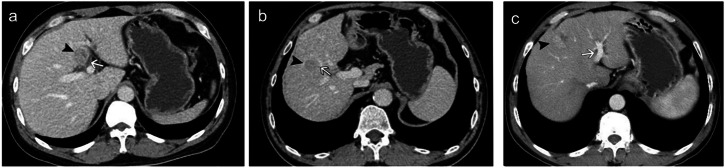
Images of peribiliary HCC (a, b) and non-peribiliary HCC (c). a Axial CT image of peribiliary HCC (black arrowhead) adjacent to the first-order branches of the intrahepatic bile duct (white arrow) with distance ≤ 5 mm. b Axial CT image of peribiliary HCC (black arrowhead) adjacent to the second-order branches of the intrahepatic bile duct (white arrow) with distance ≤ 5 mm. c Axial CT image of non-peribiliary HCC (black arrowhead) distal to the intrahepatic bile duct (white arrow) with distance > 5 mm
